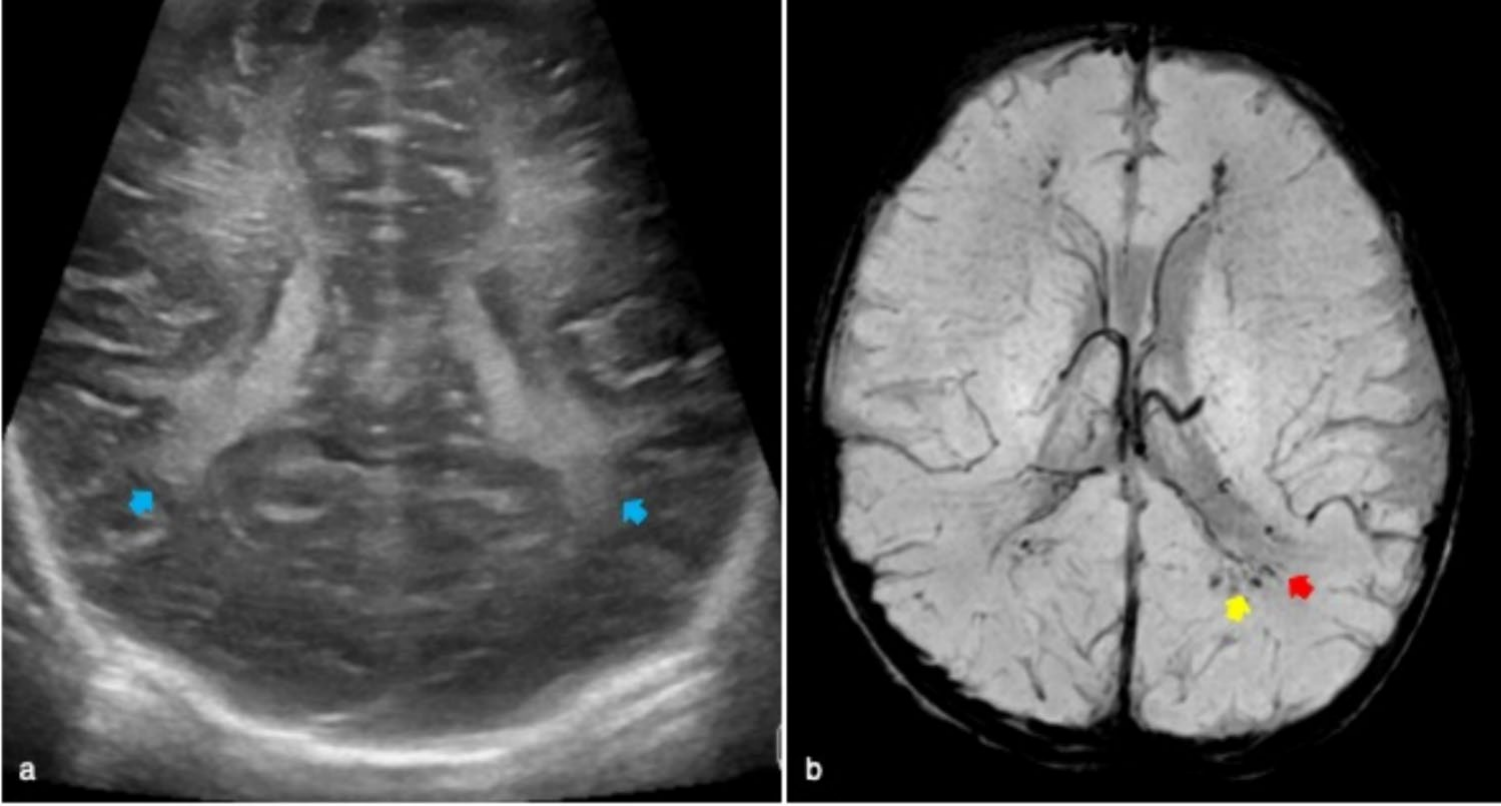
Full term male infant who underwent cerebral ultrasound at 5 days of life for severe perinatal asphyxia requiring neonatal cardio-vascular reanimation. Coronal ultrasound showed fuzzy periventricular hyperechogenic areas (blue arrows in a). MR performed to complete brain evaluation well demonstrated periventricular ‘comb’ appearance of the deep medullary veins (red arrow in b) and haemorrhagic, punctiform hypointense lesions adjacent to the occipital horns of the lateral ventricles on SWI (yellow arrow in b). These findings were suggestive of deep medullary vein thrombosis
